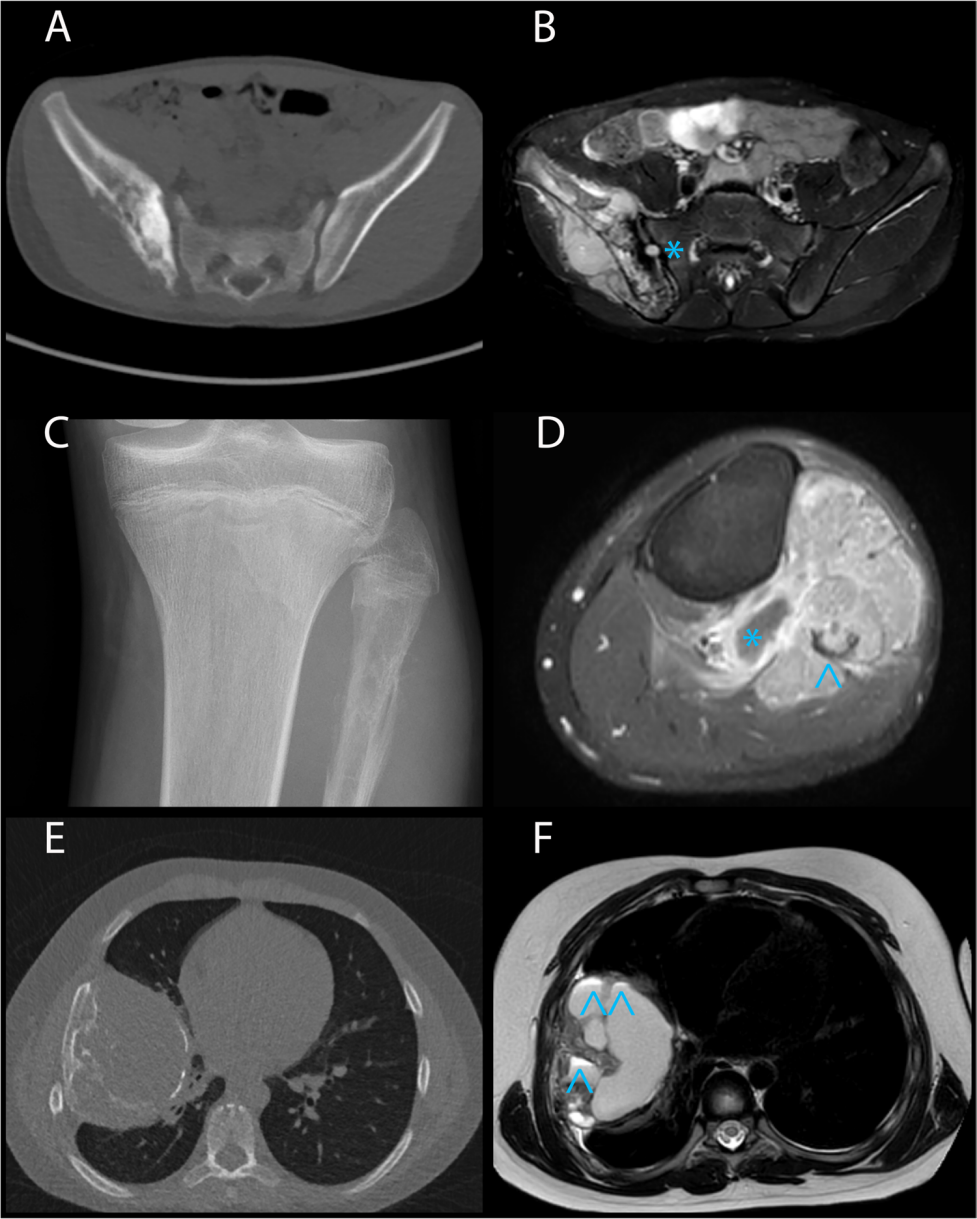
Radiology of FDRCS. A Case 12: Non-contrast pelvis CT scan reveals a permeative osseous mass in the right iliac wing with many sclerotic components suggestive for osteoid. B Case 12: Transverse T2 SPIR MR sequence shows a T2 heterogeneous mass with a large soft tissue component extending in the right iliac- and gluteal muscles. Note the T2 hypo-intense component lateral to the right SI joint resembling osteoid and the extension into the joint (T2 bright spot in the right SI joint; *). C Case 9: On the X-ray of the left knee, an ill-defined osteolytic lesion with irregular periosteal reaction in the proximal metaphysis of the left fibula is observed. D Case 9: Transverse T1 SPIR after gadolinium MR sequence depicts a rather homogeneously enhancing mass with large soft tissue component in the anterior- and deep posterior region of the proximal left lower leg. Note the small non-enhancing cystic component (*) and the osseous destruction of the proximal fibula (arrowhead). E Case 14: The non-contrast chest CT shows a rounded mass in the right hemithorax with some sclerotic components and osseous deformation and destruction of the inner cortex of the sixth right rib. F Case 14: The corresponding transverse T2 MVXD MR sequence depicts the very heterogeneous mass with both homogeneous T2 hyperintense and T2 hypo-intense components. Note the 3 small fluid–fluid levels in the hemorrhagic cystic components (arrowhead)
